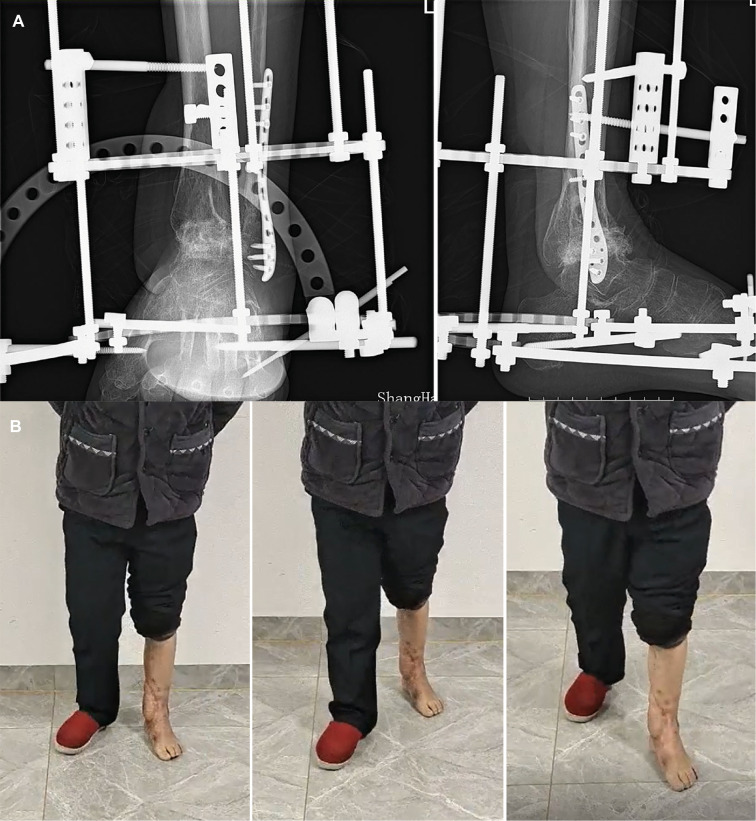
(A) The radiograph shows fracture healing 16 weeks postoperatively. (B) Appearance and gait at the 22-month follow-up.
